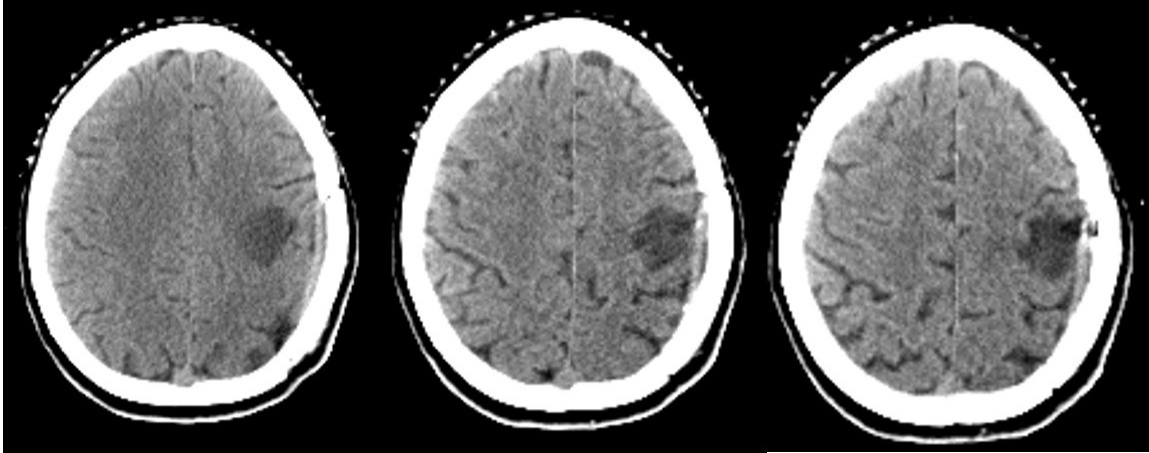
^18^FDG-PET/CT showing no FDG uptake in the region of the resected brain mass
